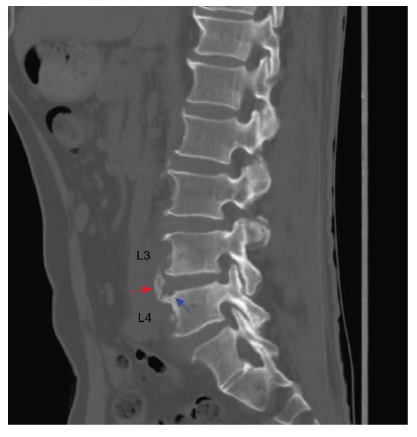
Computed tomography scan (sagittal section) showing focal erosion at the anterior superior corner (blue arrow) (known as Pedro Pons’ sign) of the L4 vertebra with prominent osteosclerosis and osteophytes (red arrow) resembling “a parrot’s beak”.
